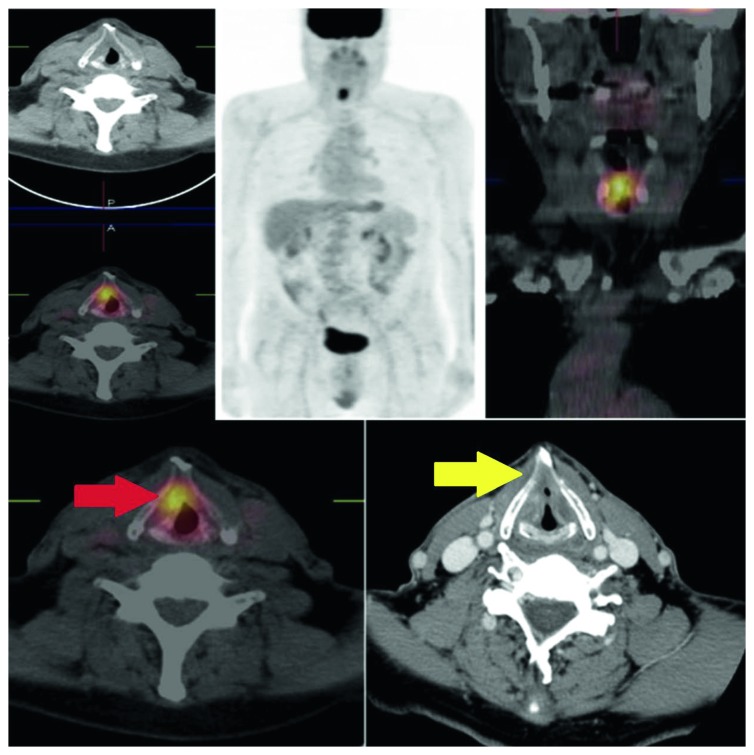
Pt T4 (cartilage infiltration): PET/CT negative (FN, red arrow) vs. CECT negative (FN, yellow arrow). SUVmax, 9.46; MTV, 2.35 cm^3^.
